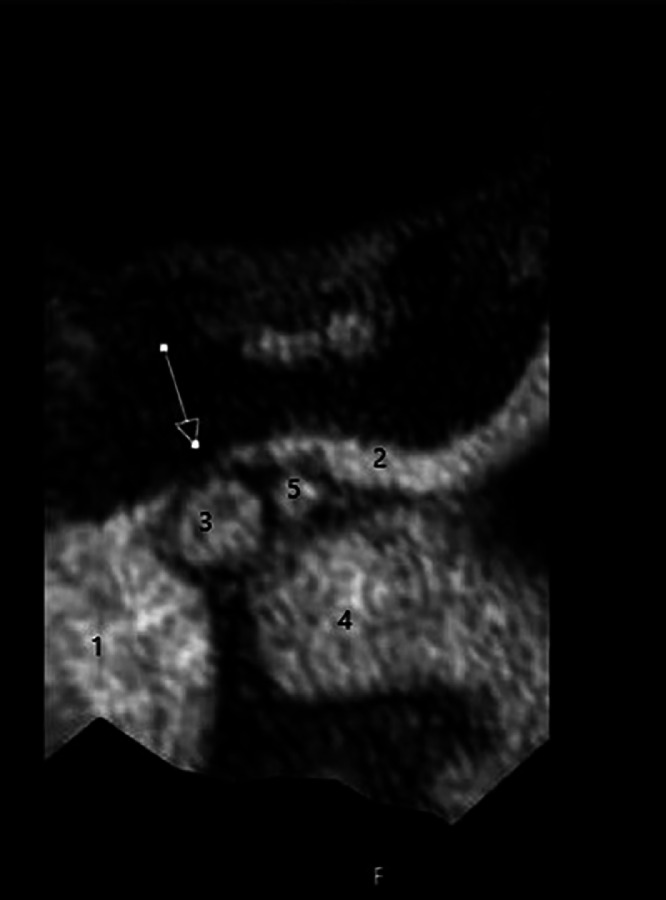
Axial section: Compression of the left brachiocephalic vein through the brachiocephalic trunk. Contrast neck angio MRI (A,E) Tesla MRI, 4D TRAK Angiography (A) Vena cava superior; (B) Vena brachiocephalica sinistra; (C) Brachiocephalic trunk; (D) Aorta descendence; (E) Arteria carotis communis sinistra; Arrow: Compression of the left brachiocephalic vein through the brachiocephalic trunk.
